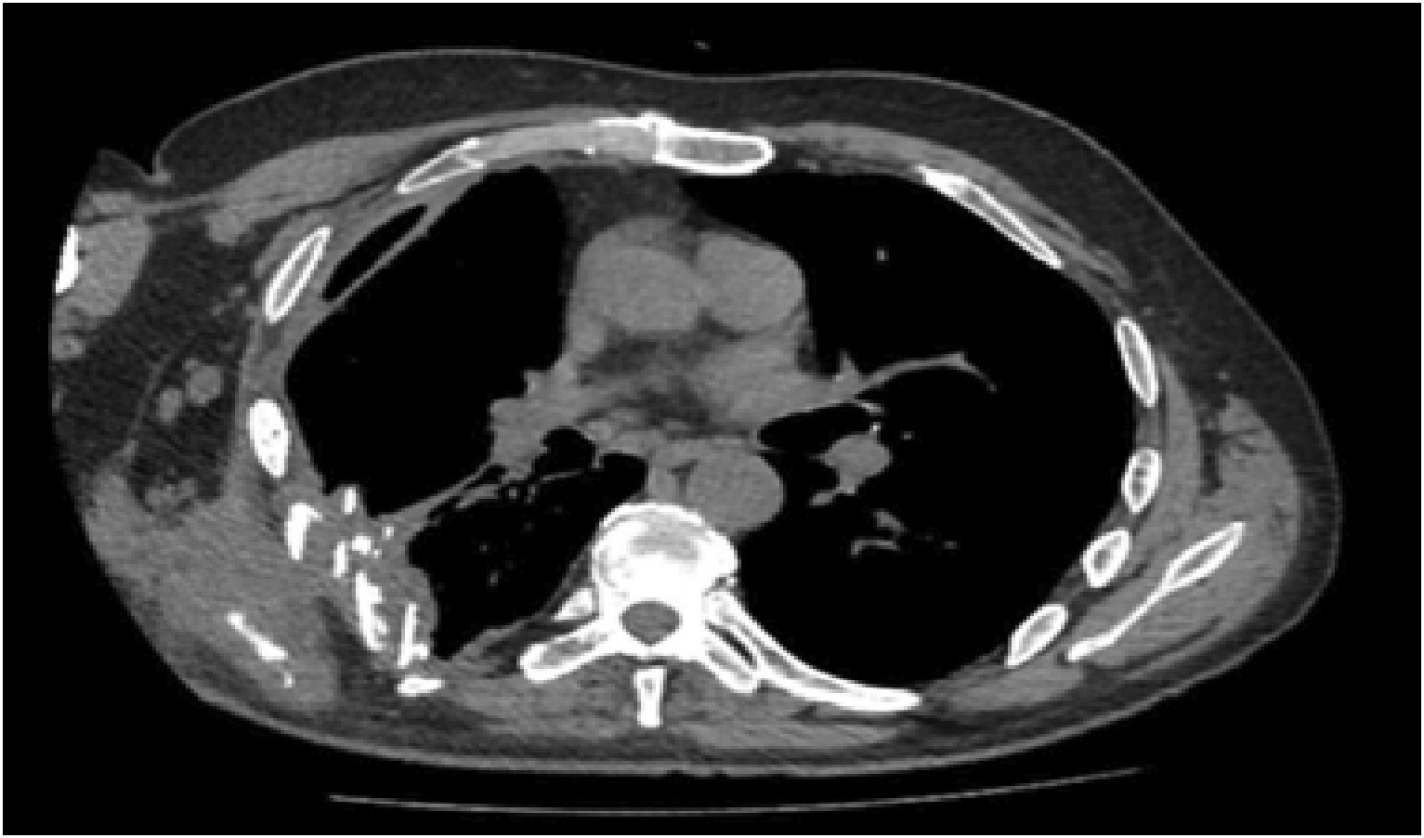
MRI scan showing chest wall deformity and rib fractures
